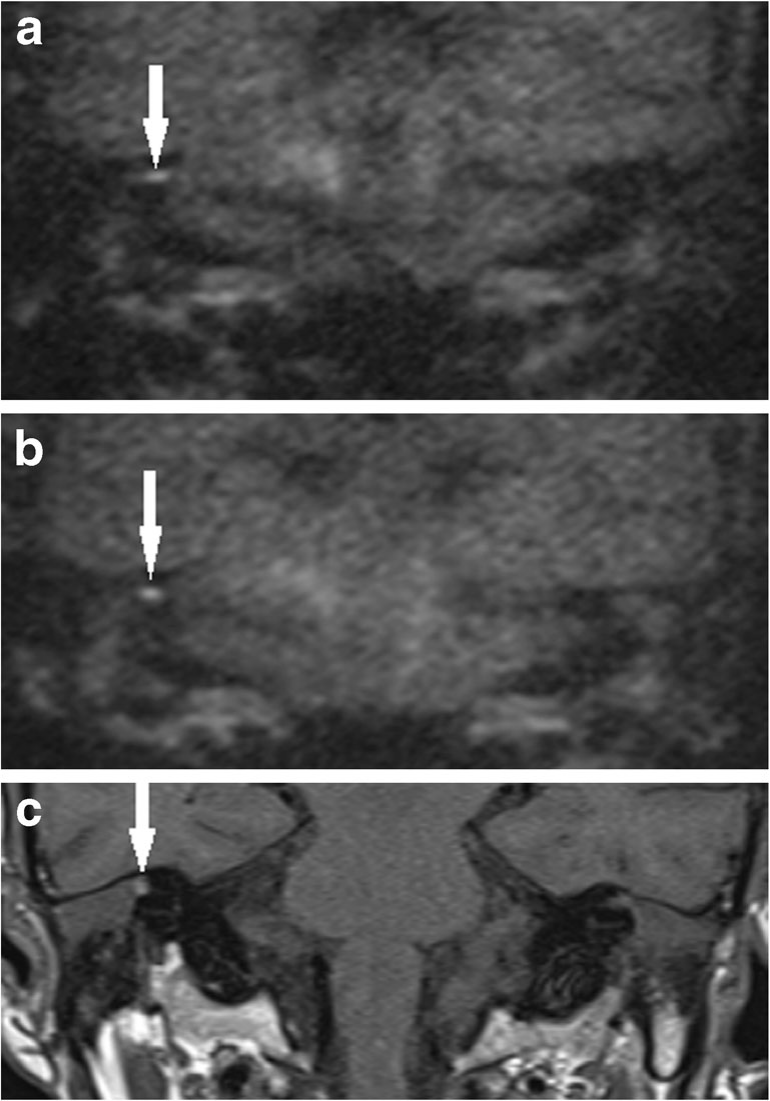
False positive case of proteinaceous fluid in the mastoid remnant. The coronal a b1000 image shows a small focus of high signal (*white arrow*) postero-medially at the mastoid remnant, which returned low signal on the ADC map, but was distant from the site of the original middle ear cholesteatoma which was excised with high surgical confidence. At the clinico-radiological meeting, it was decided to monitor this annually with DWI. b The subsequent coronal b1000 image showed the high signal focus (*white arrow*) remained unchanged over 2 years making it more unlikely to represent residual disease. c The corresponding non-contrast enhanced T1 weighted image showed the lesion (*white arrow*) returned high T1 signal suggestive of proteinaceous fluid given its stability
